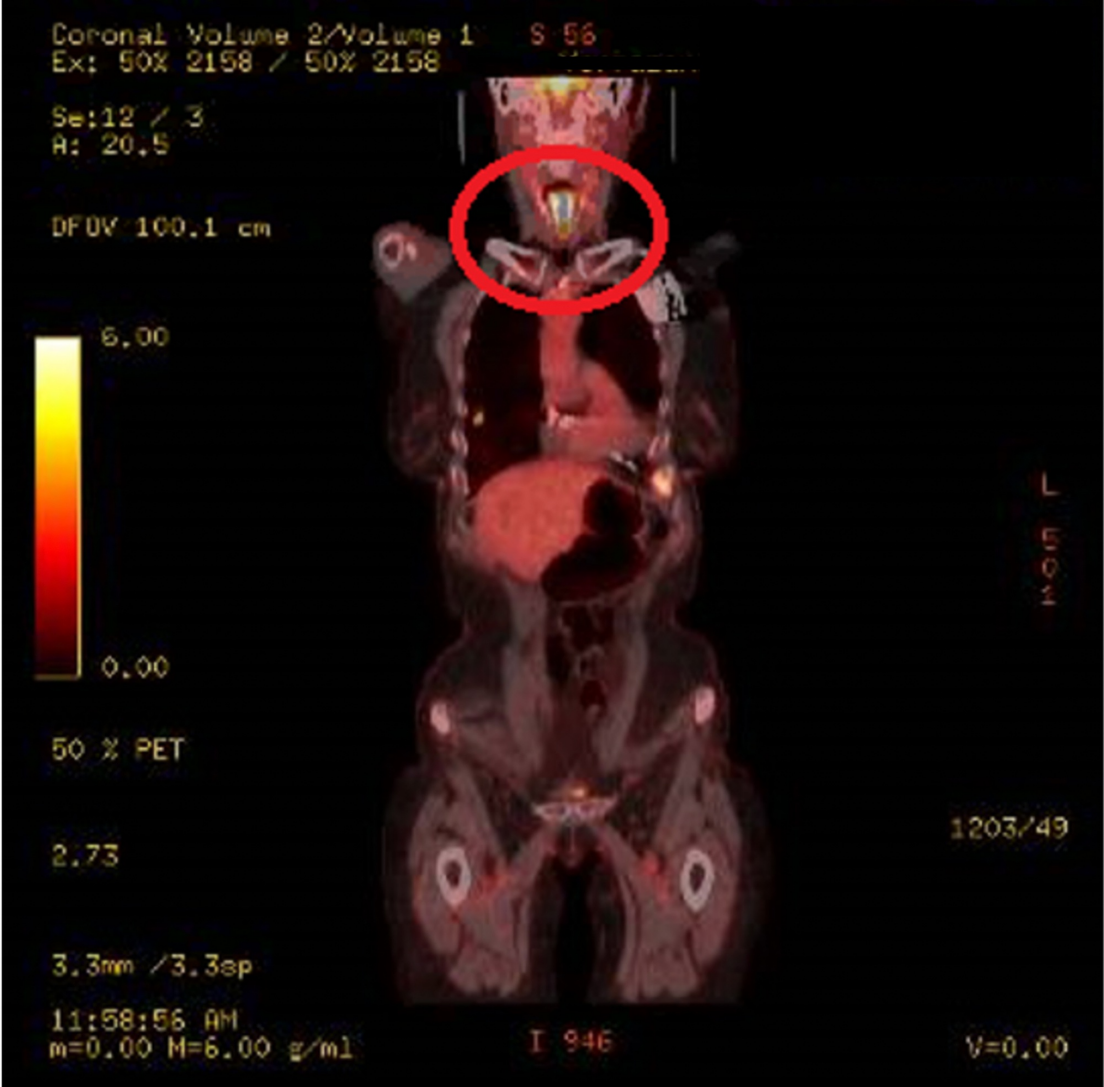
Positron emission tomography (PET) scan showing pathologic fluorodeoxyglucose (FDG) uptake within the larynx and trachea with extension into the left mainstream bronchus as well as the proximal left upper and lower lobe bronchi. The involvement of bilateral cervical chain lymph nodes was also noted. Diffuse standardized uptake value (SUV) was greatest in the larynx (20.5) (red circle).
